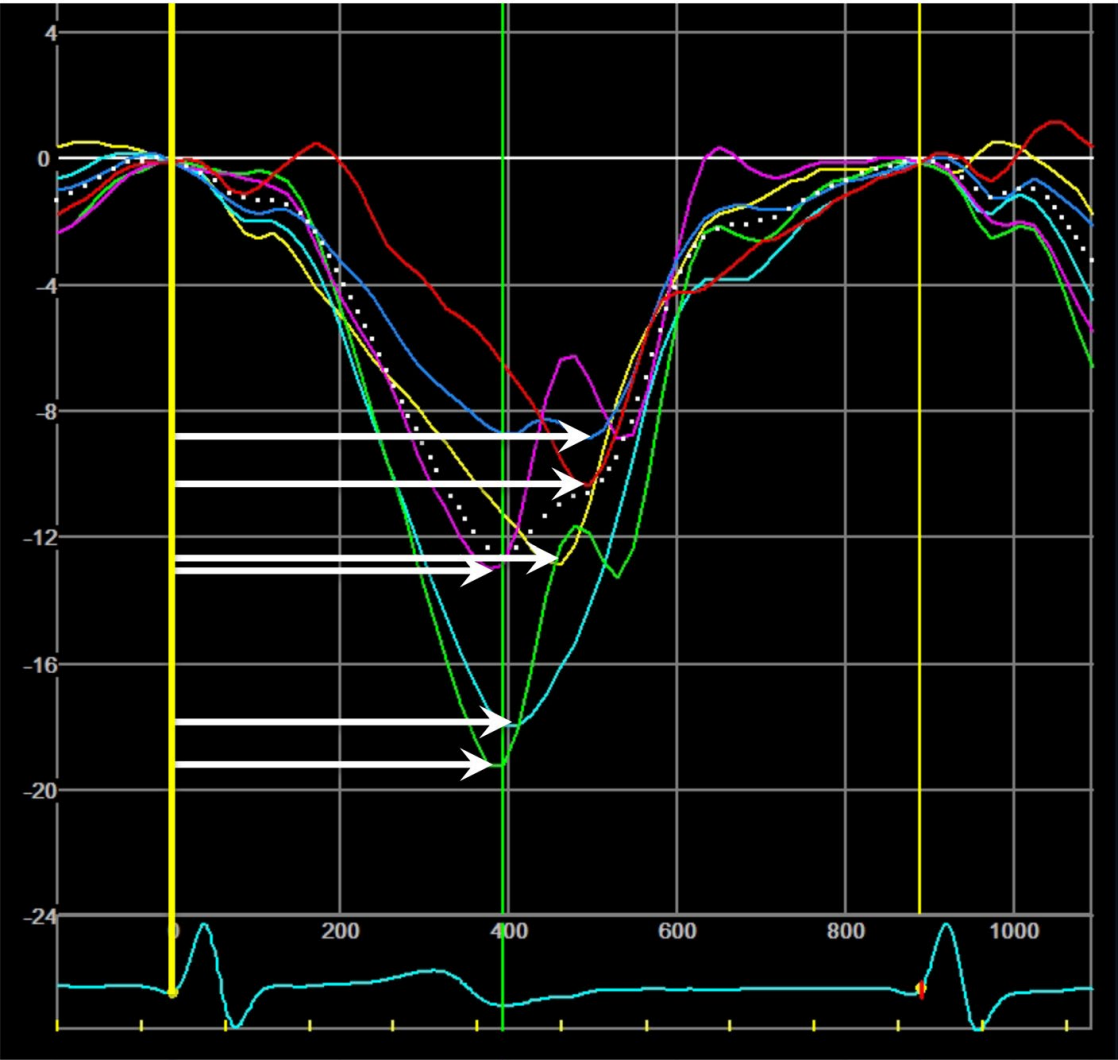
Measurement of mechanical dispersion from longitudinal strain analysis. Time-to-peak strain (horizontal arrows) was measured in each myocardial segment derived from 2D speckle-tracking echocardiography. The yellow vertical line represents the beginning of Q/S, i.e. the systole. Mechanical dispersion was calculated as the standard deviation of time-to-peak strain in 17 myocardial segments
